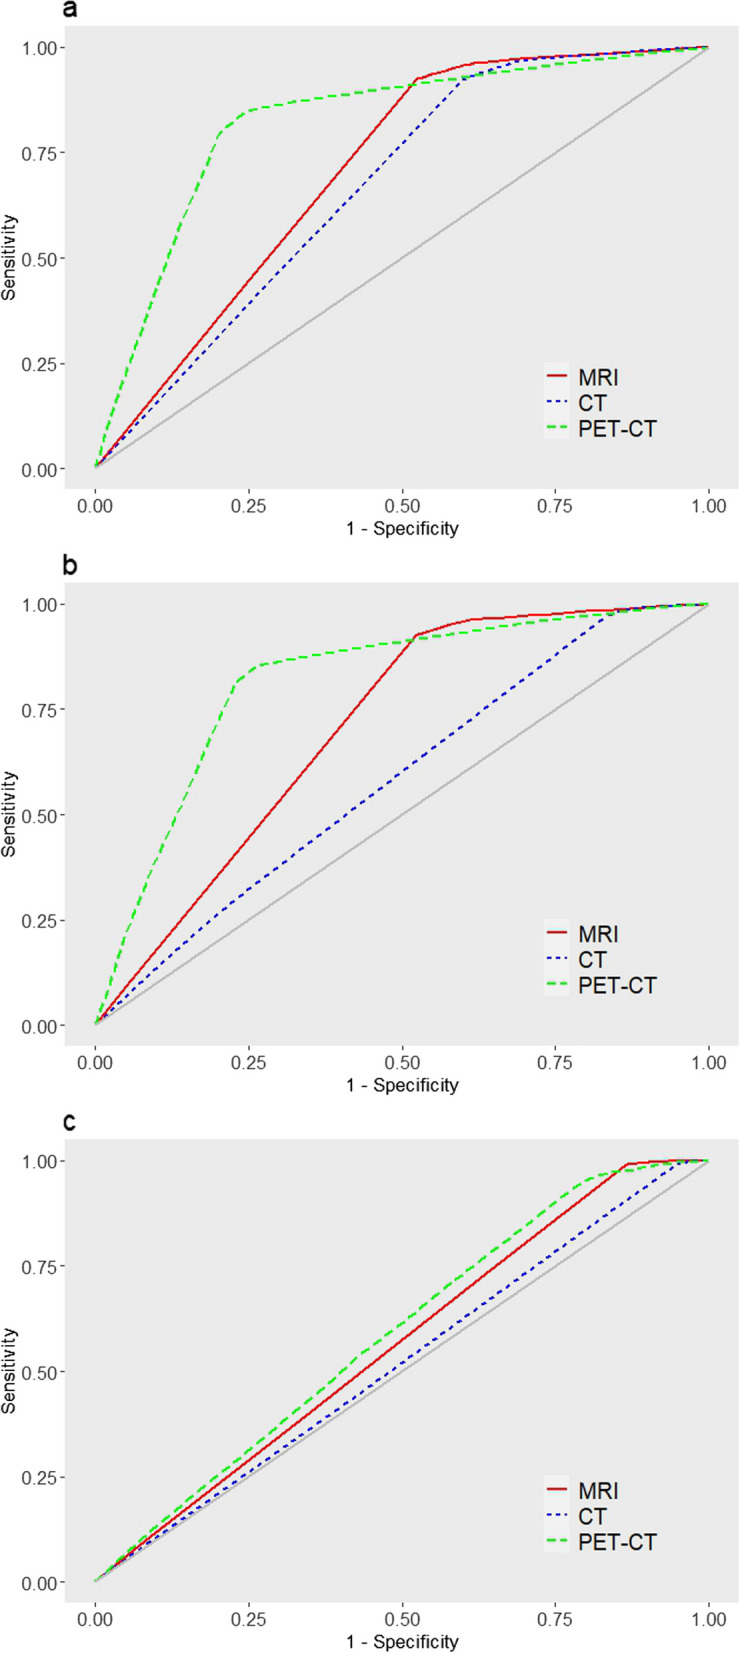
ROC-curves for MRI, CT, and [^18^F]FDG-PET-CT in detecting lymph node metastases regarding (a) patient-based, (b) region-based pelvic, and (c) region-based common iliac analyses
